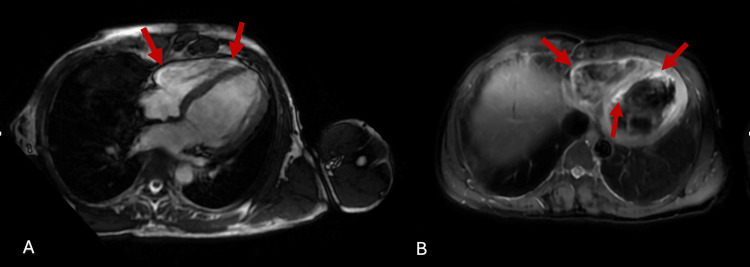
Cardiac MRI - Red arrows indicate right ventricular wall, apical (A and B), and septal (B) segments fibrosis and myocardial edema. MRI - Magnetic resonance imaging
